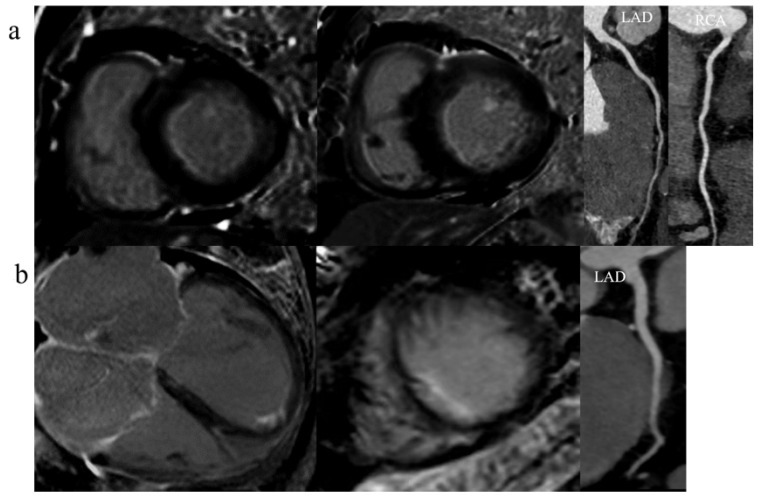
Types of SILGE: subendocardial linear LGE (a); subendocardial patchy LGE (b) in representative cases with normalized coronary artery confirmed with invasive CCTA. CCTA, coronary computed tomography angiography.
